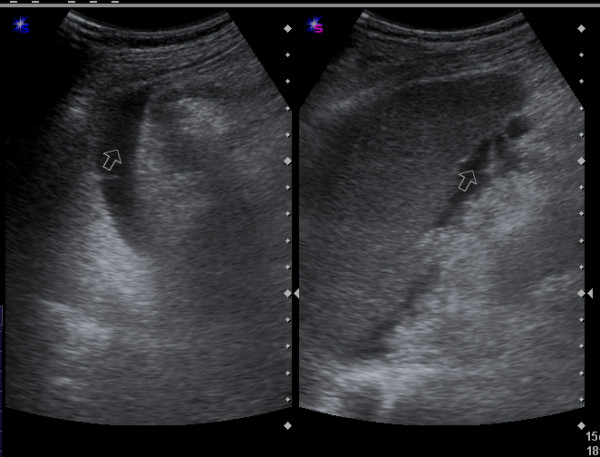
Longitudinal sonogram show free fluid (arrow) associated with Ileal perforation in pelvic cavity.
